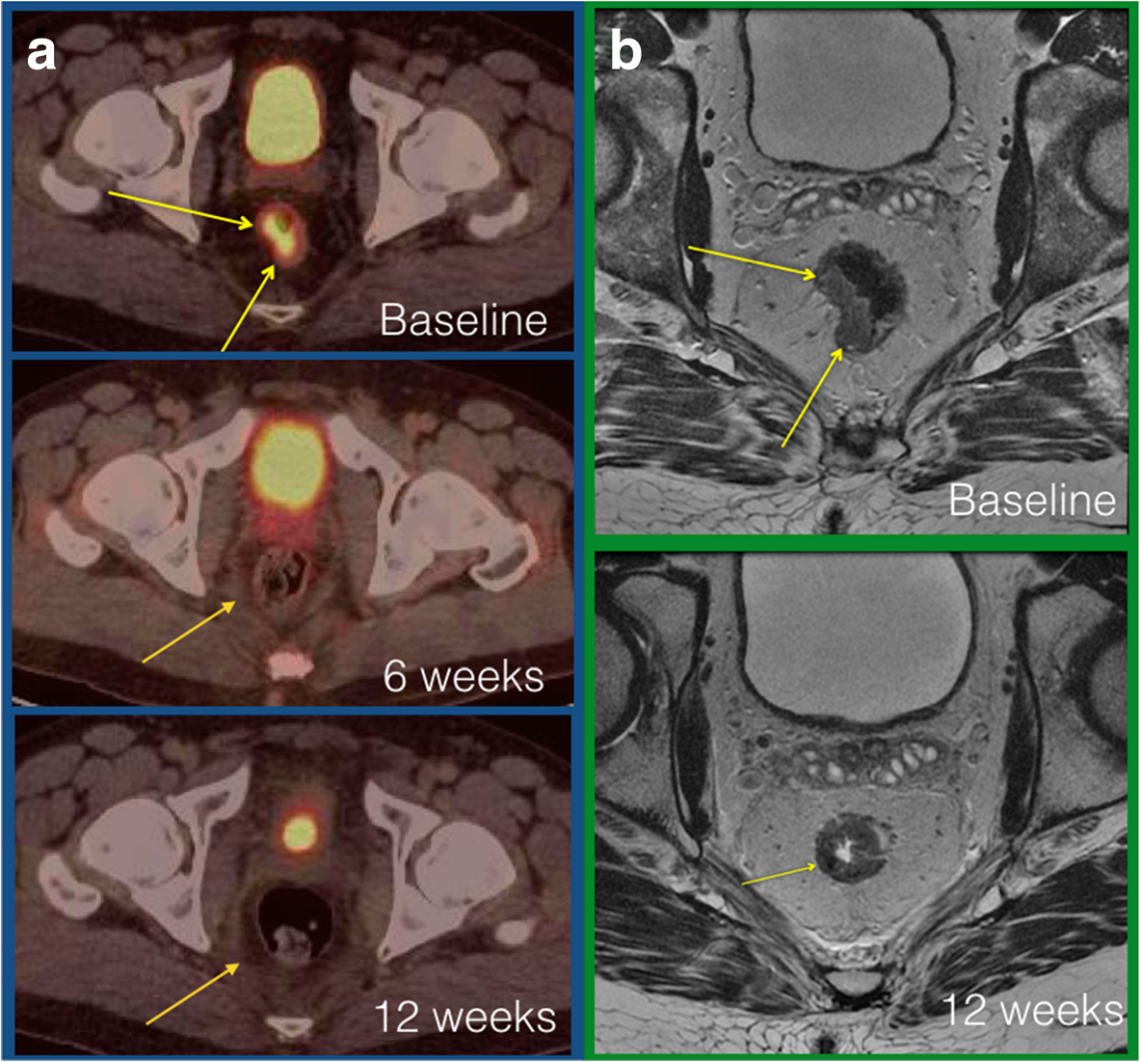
a PET/CT imaging (baseline / 6 weeks / 12 weeks) in a patient that developed complete clinical response; b MR imaging from the same patient at baseline and at 12 weeks after CRT
